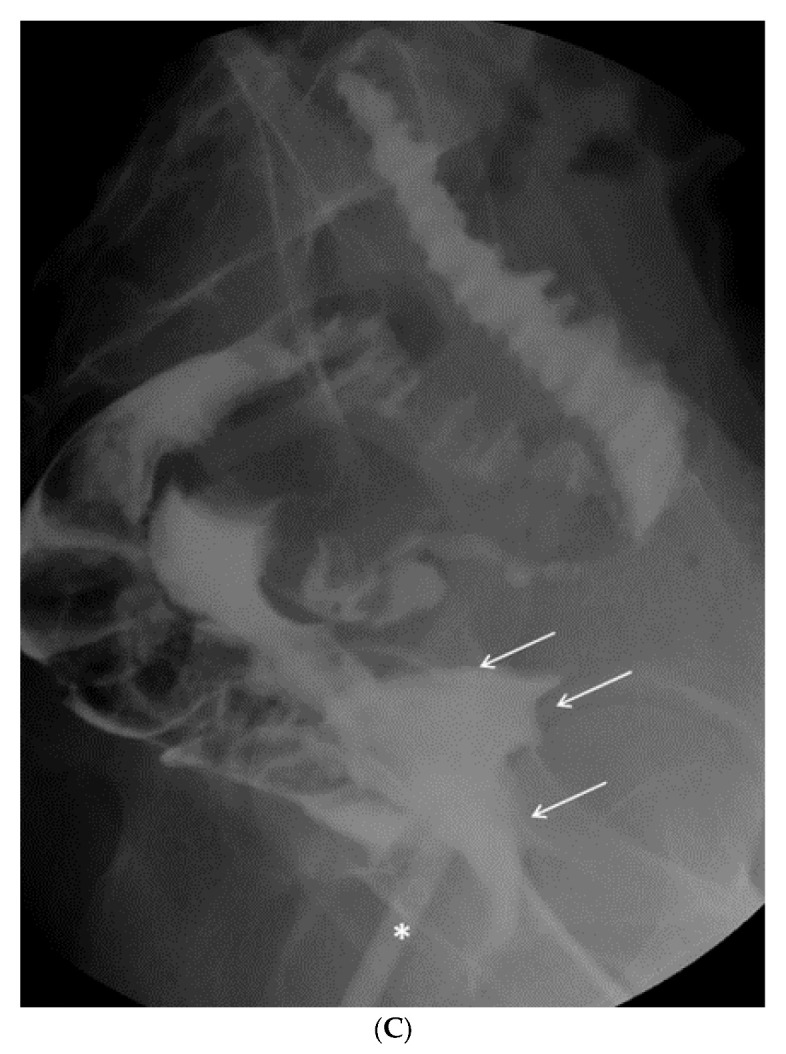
(A) Plain radiography shows free subdiaphragmatic air (white arrows). (B) Contrast-enhanced CT. A small fistula (white arrow) connects the rectum with the upper portion of the vaginal canal. Diffuse thickening of soft tissues close to the mesorectal fascia is associated with a thin fluid layer (asterisks). (C) A frame of fluoroscopy obtained through single-contrast enema performed with gastrografin. The contrast medium, injected transvaginally through a small tube (asterisk), spreads into the surrounding soft tissues (white arrows) and, to a lesser amount, into the rectum (arrowhead), confirming the presence of a pervious fistula.
